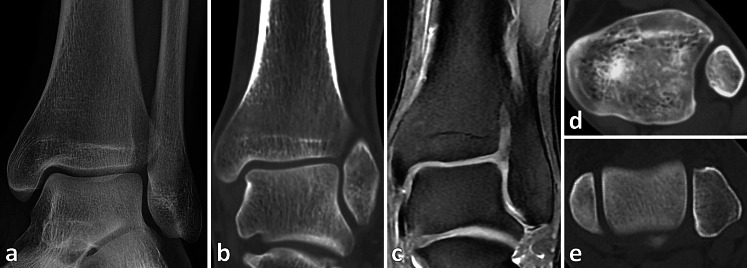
Patient N- 5. a – a mortise view; b – a frontal CT scan; c – a frontal MRI scan; d – an axial CT scan taken 5 mm proximal to the joint line; e - an axial CT scan taken 5 mm distal to joint line. Figures a, b, c and e show normal anatomy of the lateral and medial gutters, Figure c shows an intact interosseous tibiofibular ligament, Figure d documents widening of tibiofibular space of 1 mm
